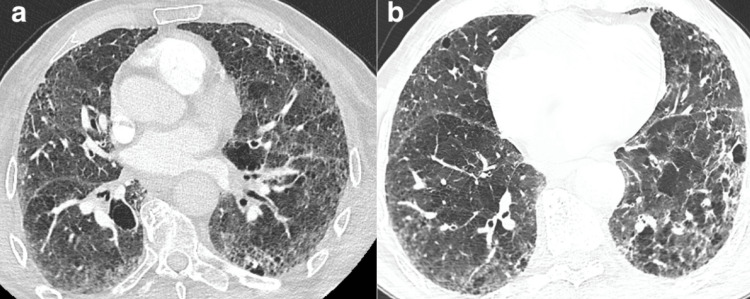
Computed tomography (CT) scan of lungs (a, b) Two-level thoracic CT scans showing lung emphysema.
